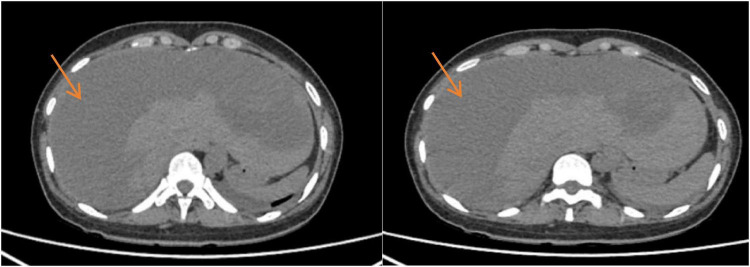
Follow-up abdominal CT after embolization (postoperative day 7/pre-discharge). Contrast-enhanced CT performed 7 days after transcatheter arterial embolization showed a slight reduction in the size of the right-lobe subcapsular hematoma (arrow) with decreased attenuation compared with admission. No new contrast extravasation, expanding hemoperitoneum, or progressive intraperitoneal fluid collection was observed, indicating successful hemostatic control.
